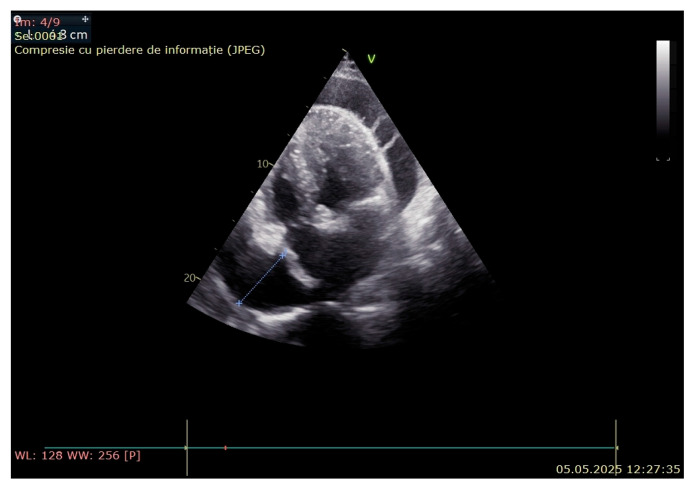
Echocardiography revealed right atrium collapse and massive pericardial fluid.
